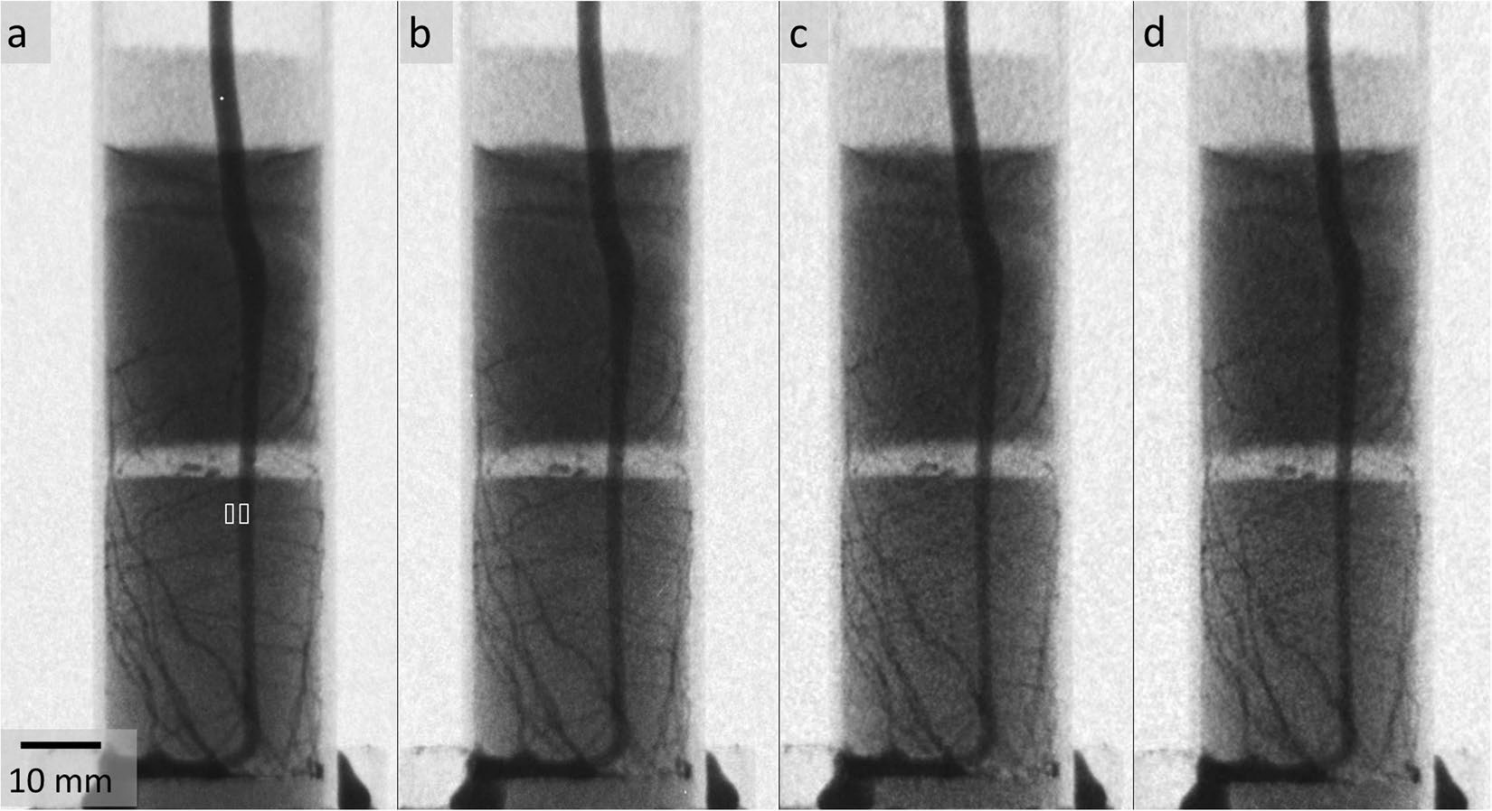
Analogous radiographic projections captured while sample was rotating in four different ultra-fast neutron tomographies constituting a tomographic test series. (a) 60 s – tomography: t_exp_ = 0.2 s; (b) 30 s – tomography: t_exp_ = 0.1 s; (c) 15 s – tomography: t_exp_ = 0.05 s; (d) 10 s – tomography: t_exp_ = 0.05 s. The white rectangles in a indicate regions used for contrast calculation.
